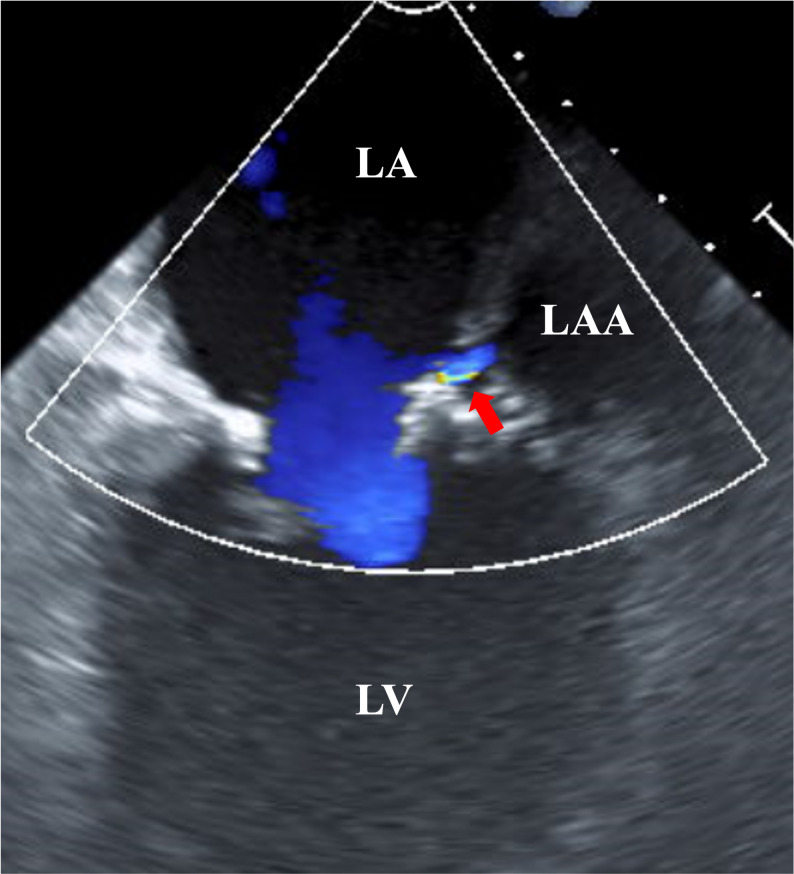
Intraoperative transesophageal echocardiography. TEE demonstrating residual flow (arrow) in a patient who underwent left atrial appendage occlusion with a single-layer purse-string suture. The arrow indicates persistent flow from the LAA to the LA cavity. *TEE* transesophageal echocardiography, *LA* left atrium, *LV* left ventricle, *LAA* left atrial appendage
